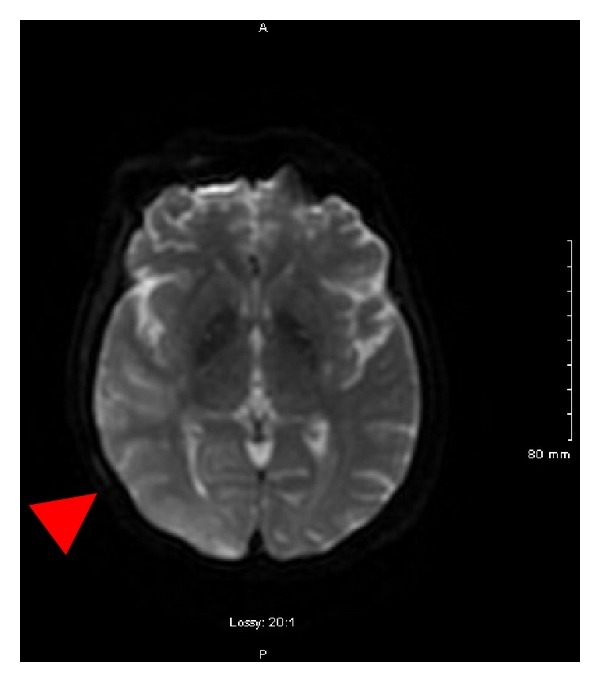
MRI of the brain showing a large area of gyral edema, sulcal effacement, and cortically based diffusion restriction involving the right occipital lobe and right posterior temporal and parietal lobes (indicated by RED arrow).
